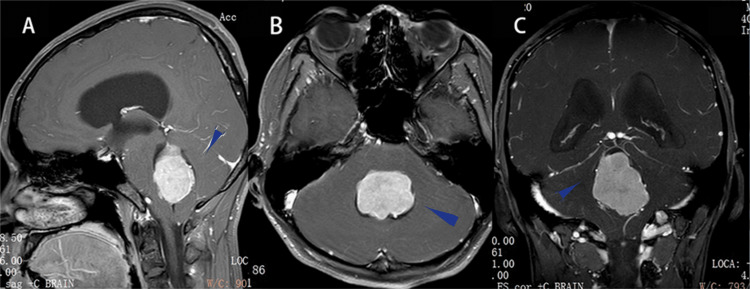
Preoperative magnetic resonance imaging T1 enhancement scan (A-C) The arrow is pointing toward the lesion.
